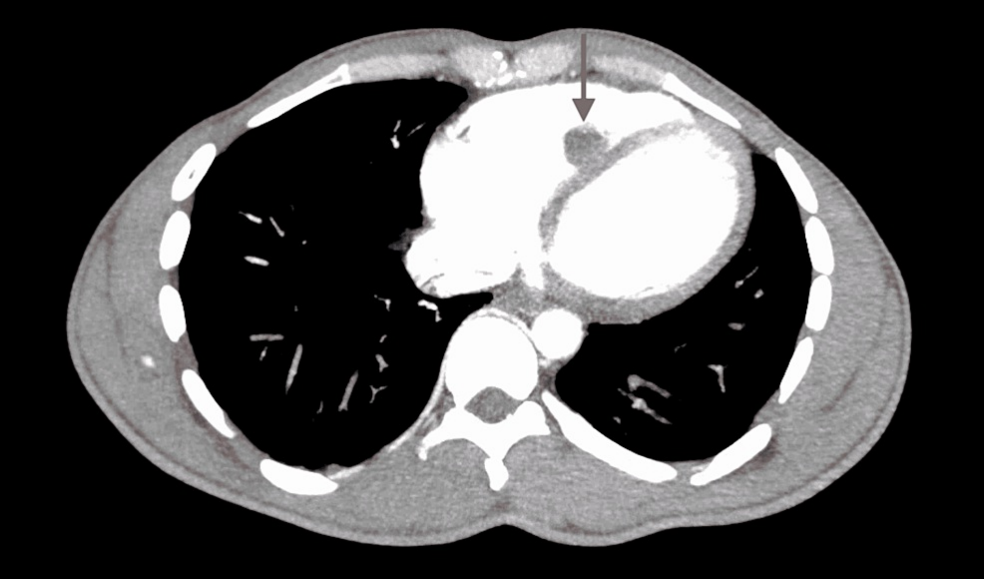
Computed tomography angiography of the chest, axial view The red arrow shows a 1.6cm rounded hypodensity within the right ventricle.
